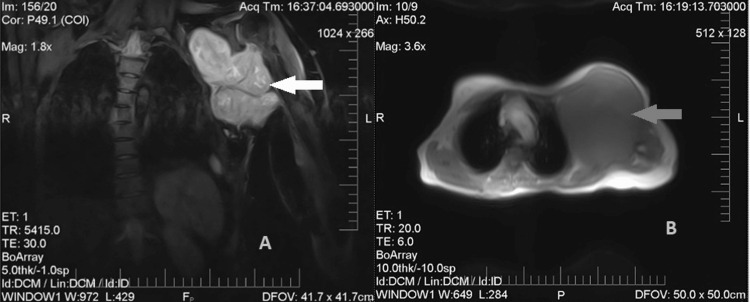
MRI chest coronal (A) and axial (B) views showing a heterogeneous large mixed signal intensity mass in the left axilla shown by white arrowhead in image A and gray arrowhead in image B
